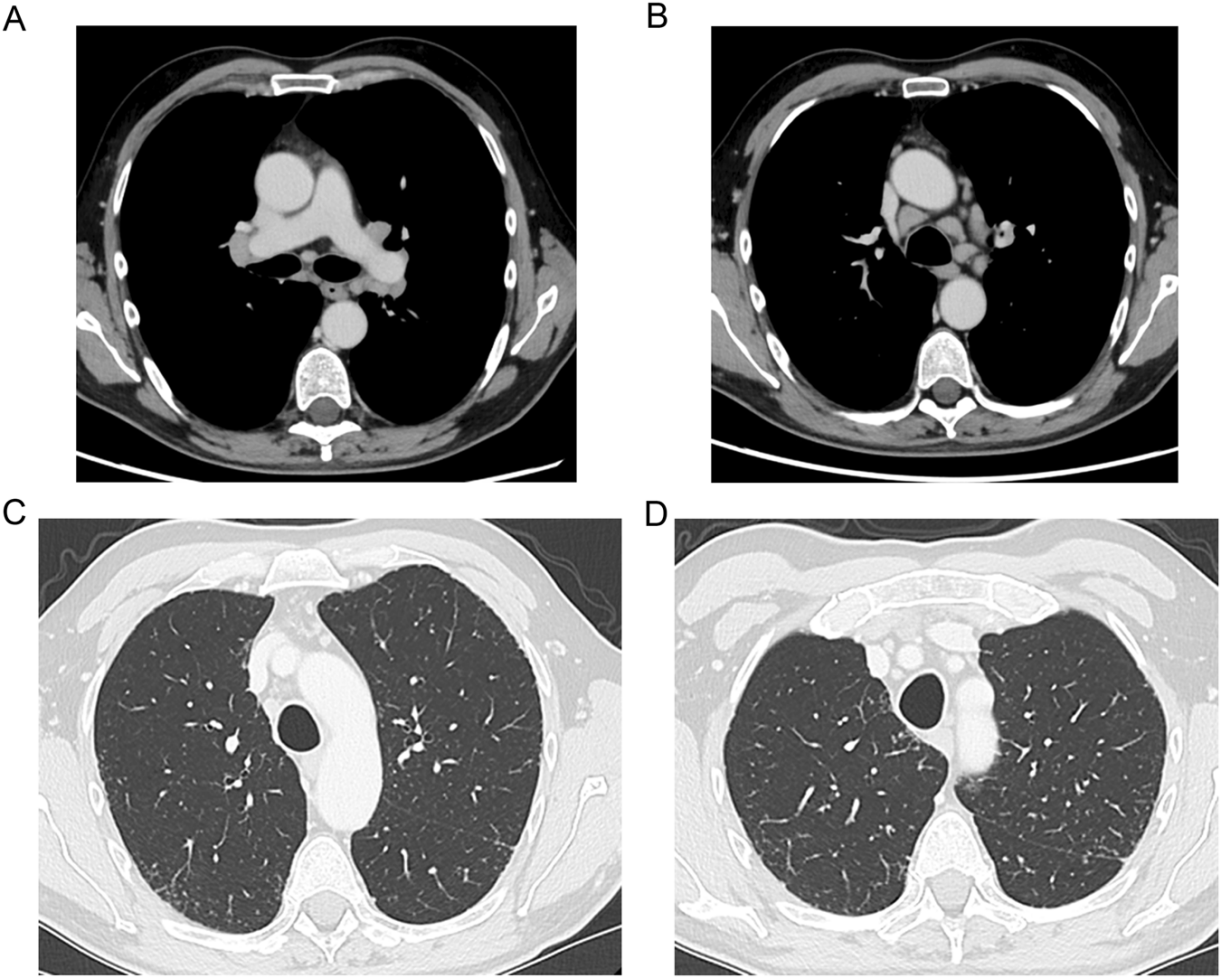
CT scans of a patient with a head and neck tumor and a sarcoid-like reaction induced by systemic anticancer therapy (diagnosed by multidisciplinary team consensus). A, B After two cycles of pembrolizumab treatment, the patient developed new mediastinal lymphadenopathy and (C, D) a new pulmonary peri-lymphatic micronodular pattern. Sarcoidosis was found in a mediastinal lymph node sample and the case was finally classified by the interstitial lung disease board as a sarcoid-like reaction
